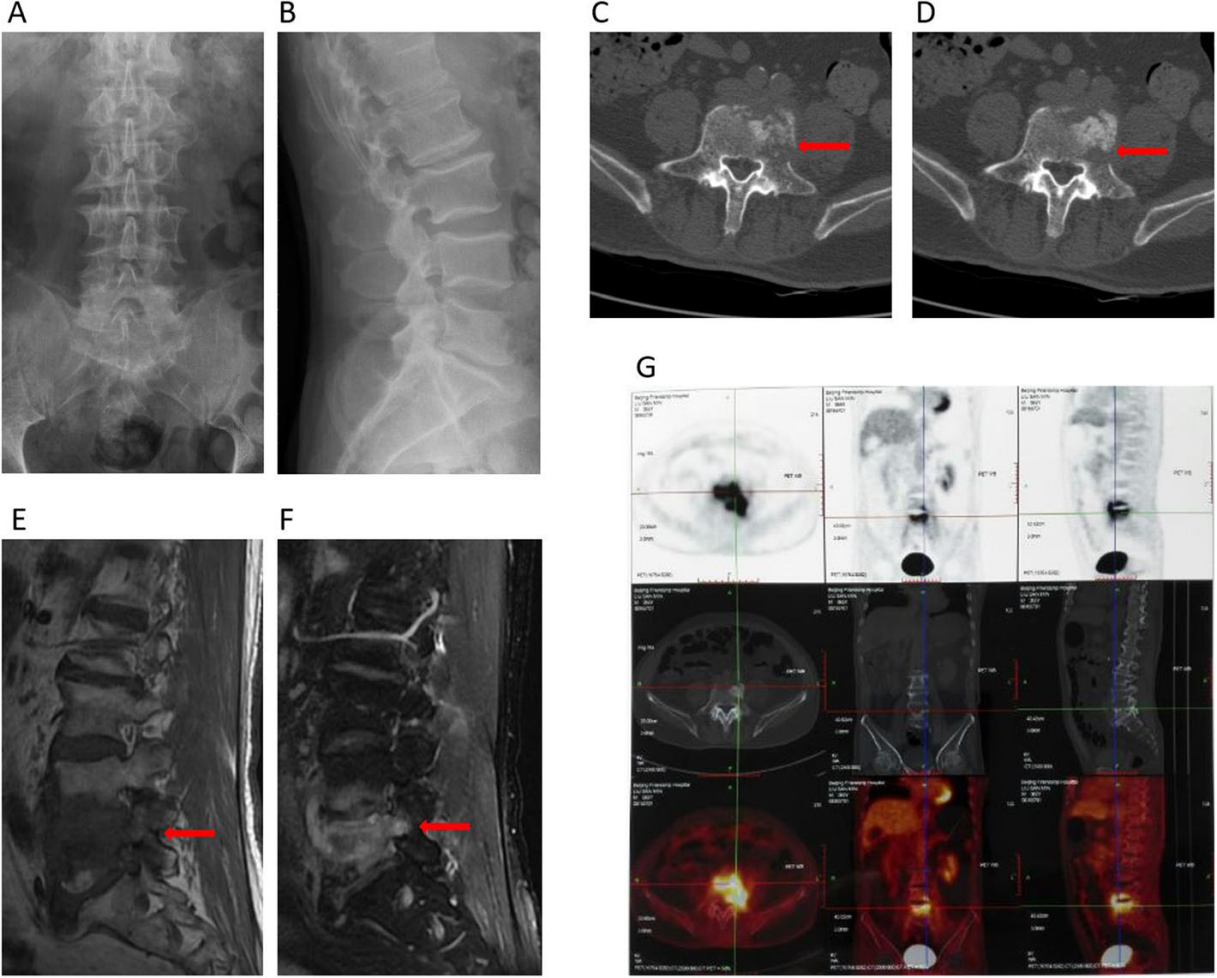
Radiological findings before treatment. Notes: (a and b) X-ray before treatment showed degeneration of lumbar spine, hyperplasia of vertebrae, no significant narrowing of L4–5 inter-vertebral space. (c and d) CT showed vertebral worm-eaten-like damages (arrow), the formation of sequestrum, hyperplasia of vertebrae. (e and f) MRI showed hypointense on T1-WI and heterogeneous hyperintense on T2-WI (arrow) in L4–5 level of vertebrae and disc. (g) PET-CT showed the significant uptake of the radioactive FDG in L4–5 level, high suspicion of infection
